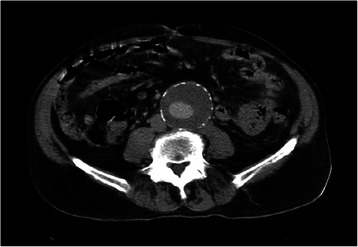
Abdominal CT with contrast: partially thrombosed aneurysm of the abdominal aorta.
